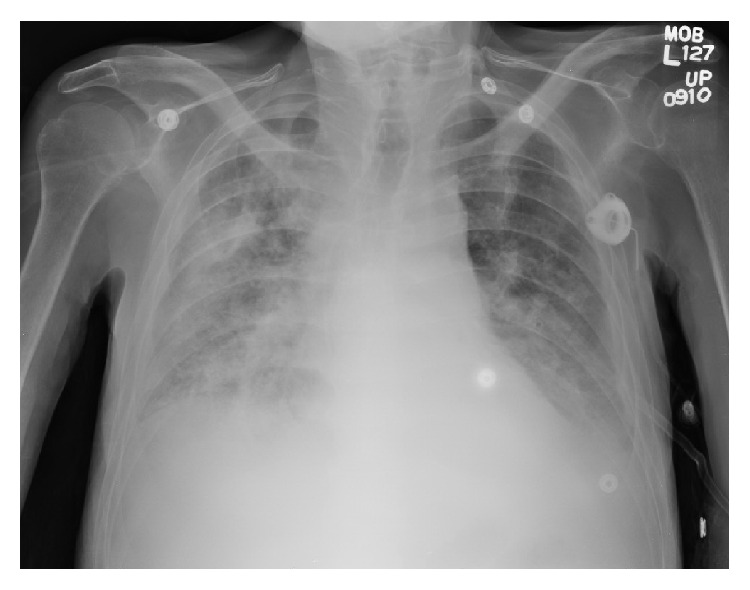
Chest X-ray showing diffuse consolidation throughout the right lung.
